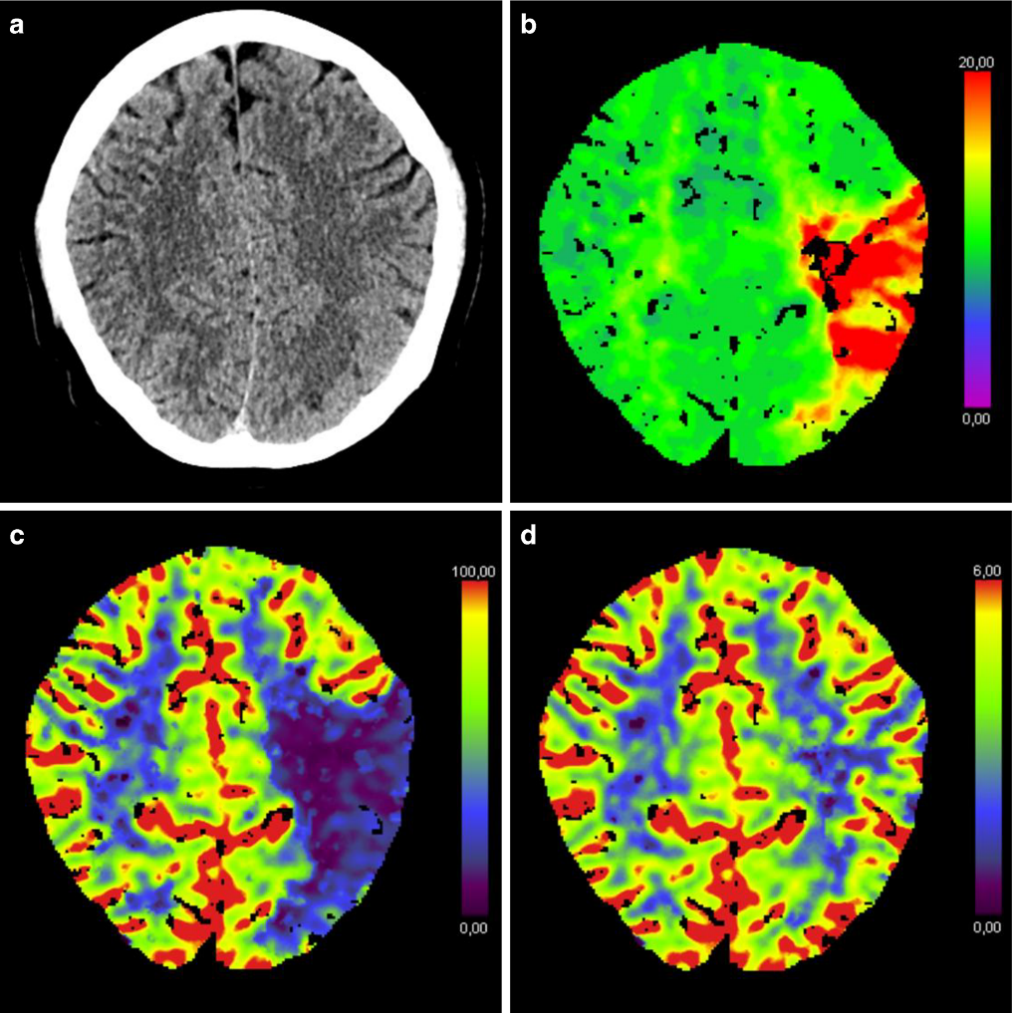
Unenhanced brain CT scan (a) shows intact corticomedullary differentiation. Contrast enhanced CT perfusion study (b, c, d) reveals elevated time to peak (b), impaired cerebral blood flow (c), and preserved cerebral blood volume (d) of the left frontal and parietal lobes including the central region
